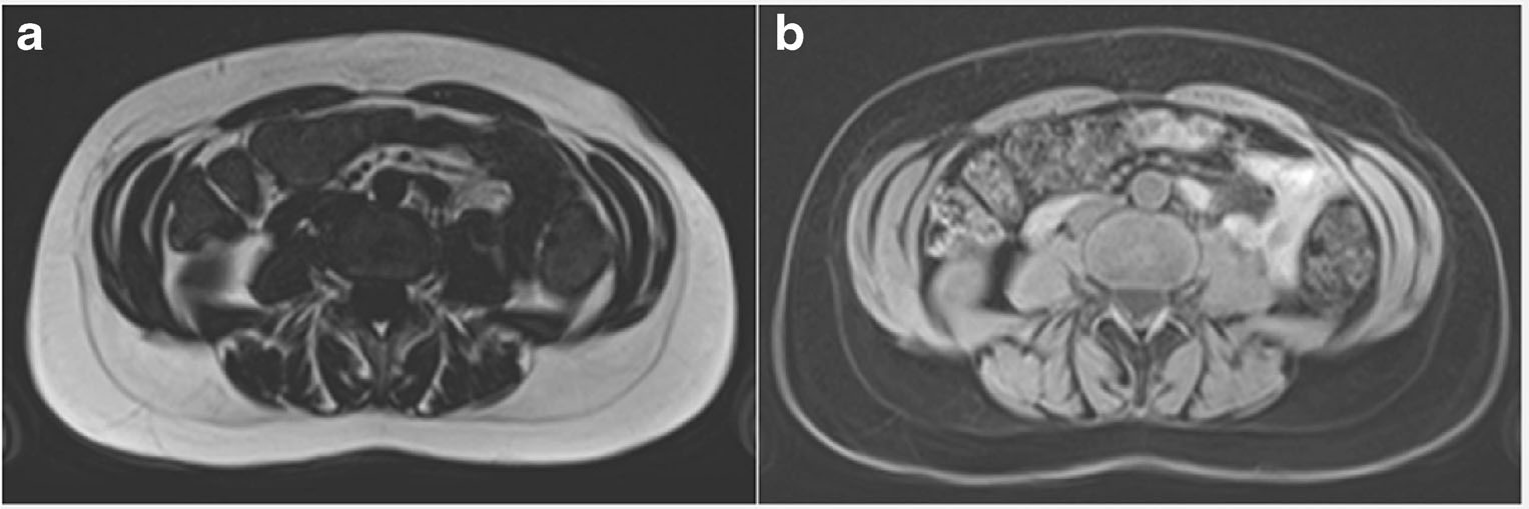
T1-weighted axial DIXON MRI images highlighting (a) fat and (b) water signals at the level of the L3 vertebral body
